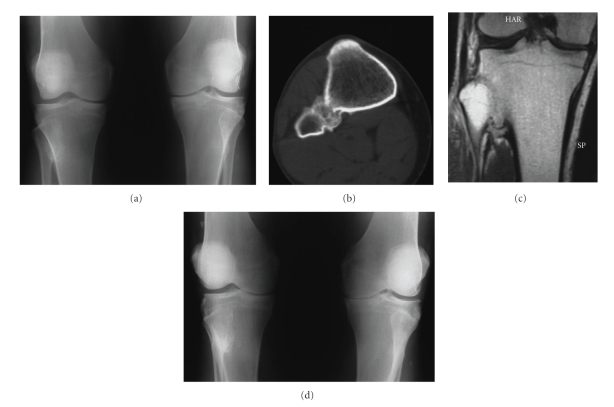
AP radiograph of both knees (a), CT (b), and MRI (c) examination, in a 55-year-old man, indicating synostosis of the proximal TFJ of the right knee initially, and 4 years later (d).
